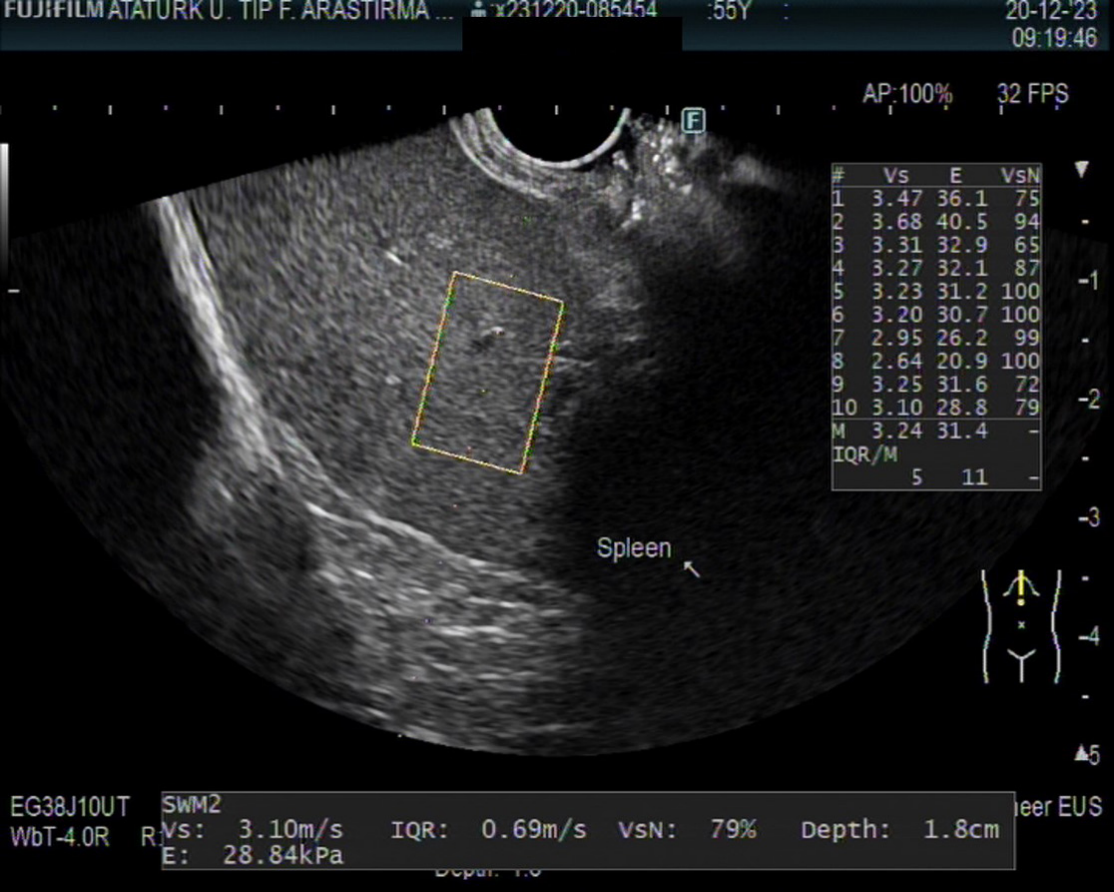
Evaluation of spleen stiffness with endoscopic ultrasound shearwave elastography.
